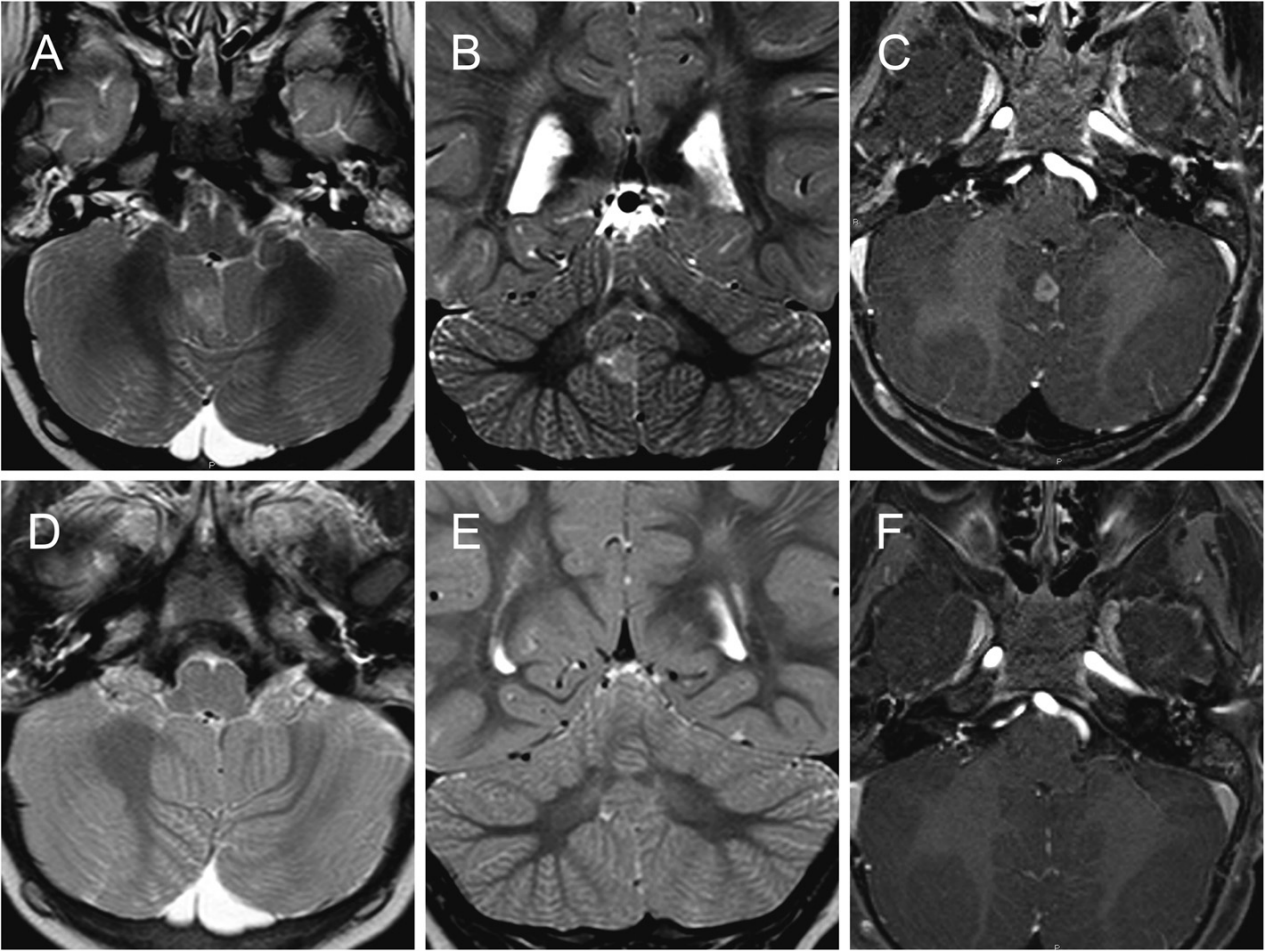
Evolution of the cerebellar lesion on brain MRI study. Axial (A,D) and coronal (B,E) T2 weighted MRI;. Axial (C,F) post-contrast T1-weighted MRI: small pseudonodular lesion on the mesial side of the right cerebellar hemisphere. The lesion was hyperintense on T2-dependent sequences (A,B) and with mild enhancement after gadolinium administration (C). Follow-up MRI performed after 6-months revealed complete disappearance of the cerebellar lesion (D-E-F).
